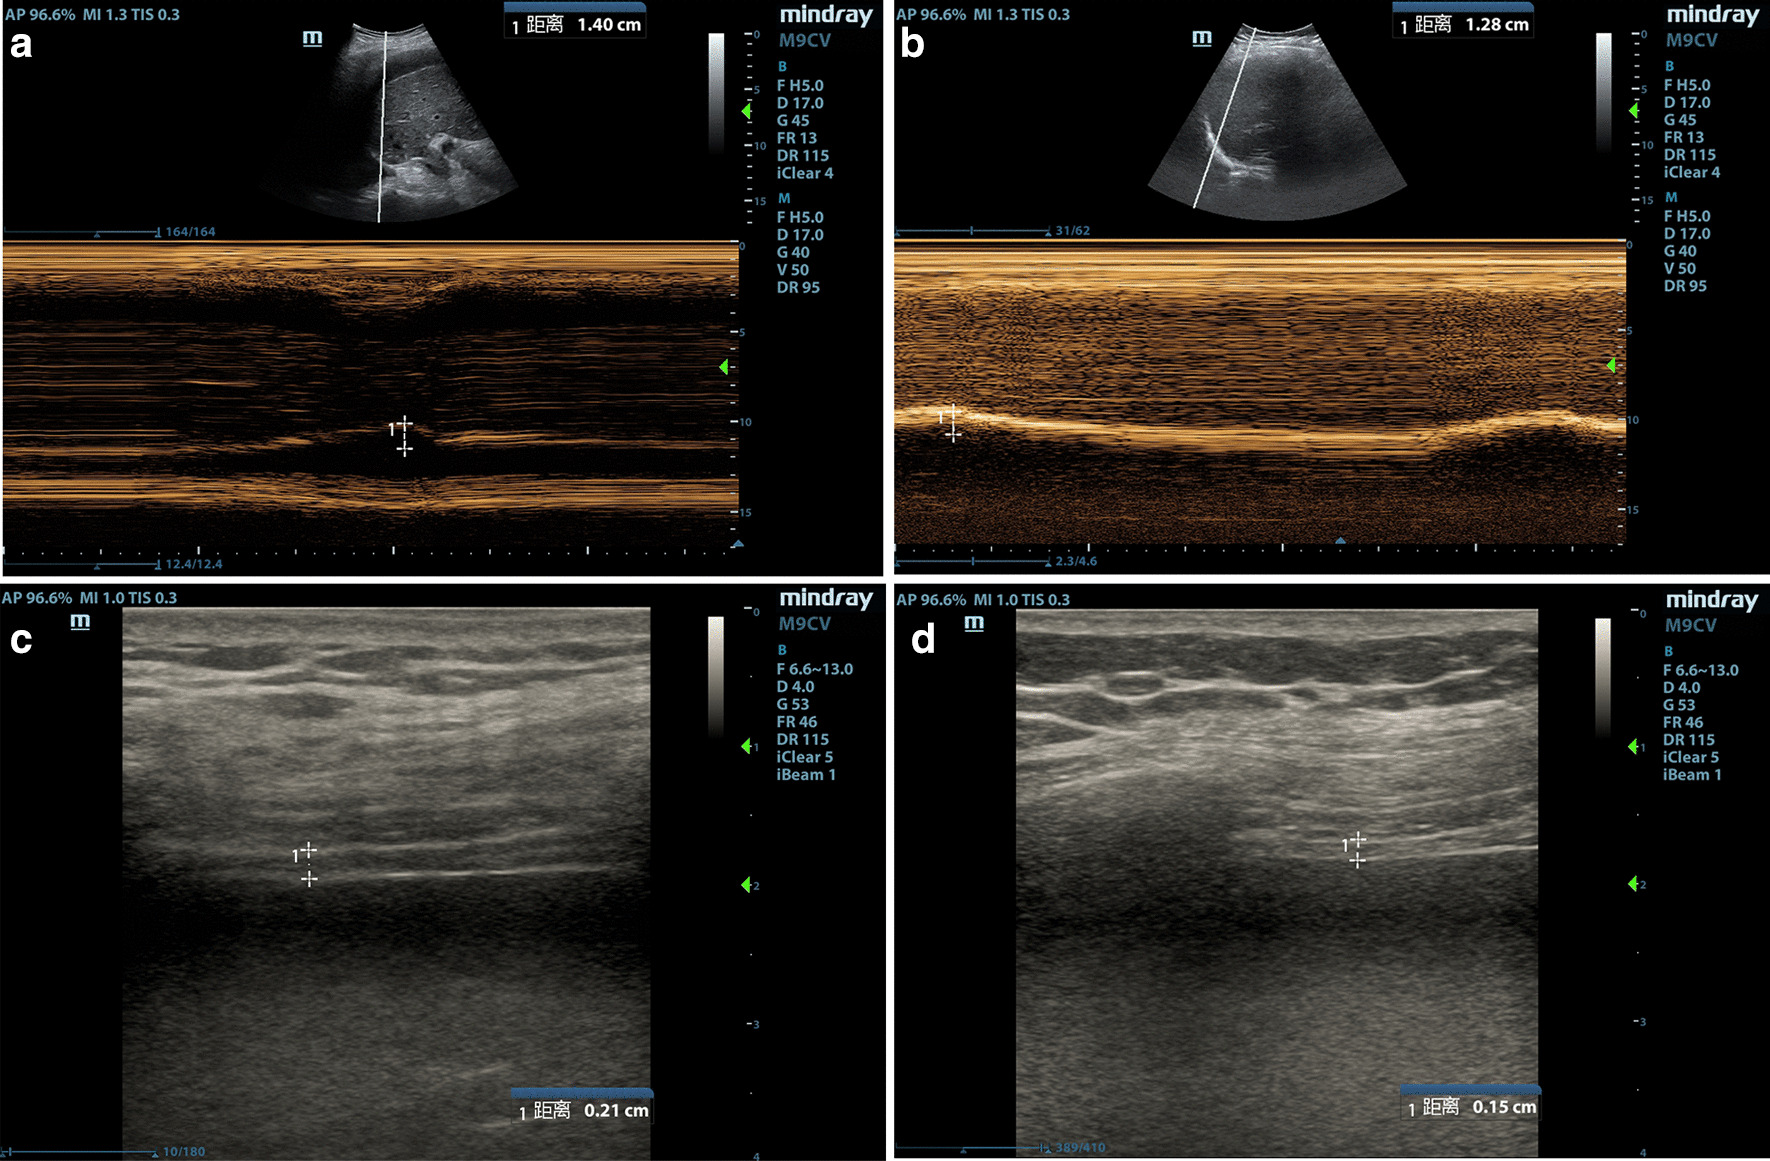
Example of ultrasonographic view before and after rehabilitation. a Diaphragmatic excursion (DE) was viewed during breathing in B-mode (upper) and M-mode (lower) before rehabilitation. b DE was viewed during breathing in B-mode (upper) and M-mode (lower) after rehabilitation. c DTF was viewed at the area of apposition before rehabilitation. d DTF was viewed at the area of apposition after rehabilitation
